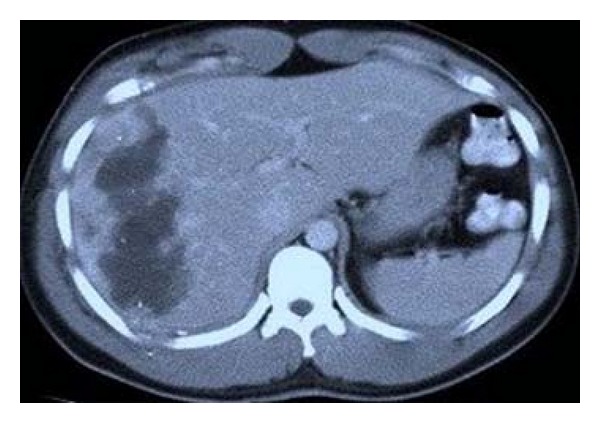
CT scan showing large hematoma in Right lobe of liver following blunt trauma. The hematoma was complicated by bilhemia.
